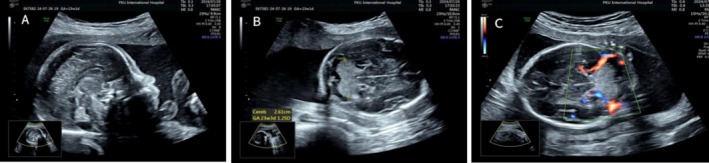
Fetal color Doppler ultrasound at 23 weeks of gestation. (A) Grayscale ultrasound of the fetal posterior fossa, showing bilateral symmetric diffuse hyperechogenicity of cerebellar hemispheres; vermis intact, no intracranial abnormalities. (B) Axial grayscale ultrasound of the fetal posterior fossa, demonstrating symmetric diffuse hyperechogenicity of cerebellar hemispheres. (C) Color Doppler ultrasound of the fetal cerebellum, revealing diffuse hyperechogenicity of cerebellar hemisphere with normal arterial and venous blood flow.
